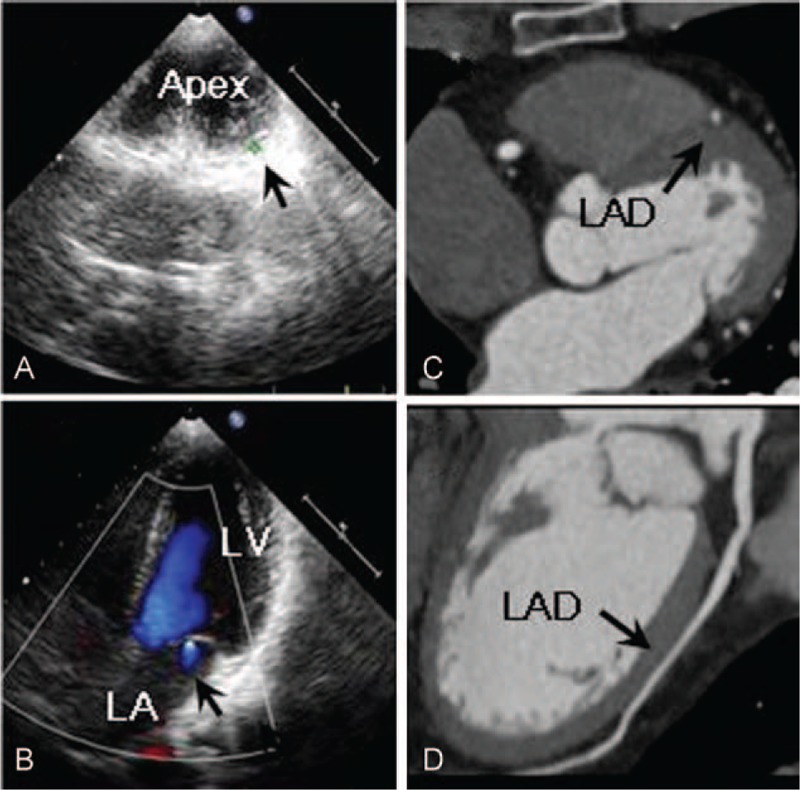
Images of echocardiograph (A–B) and cardiac CT angiography (C–D) of the patient who presents myocardial noncompaction with myocardial bridge. (A) Deep intertrabeculations recesses in the endocardial wall of left ventricular particularly in apex free wall; (B) mitral valve with regional regurgitation; (C) left descending artery (LAD) is surrounding by cardiac muscles; and (D) LAD keeps its shape against the cardiac muscles, leaving no space between the segment and cardiac muscle.
